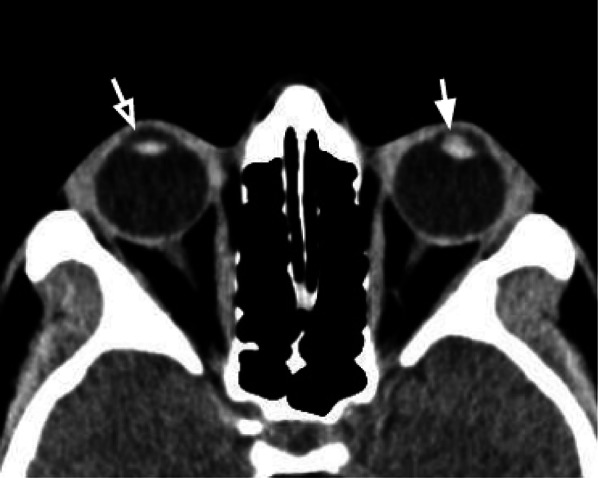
Axial CT acquisition showing a left hyphema, visible as a hyperdense (hemorrhagic) focal component in the anterior chamber, anterior to the lens (white arrow). The content of the contralateral chamber is homogeneous and hypodense (empty arrow)
